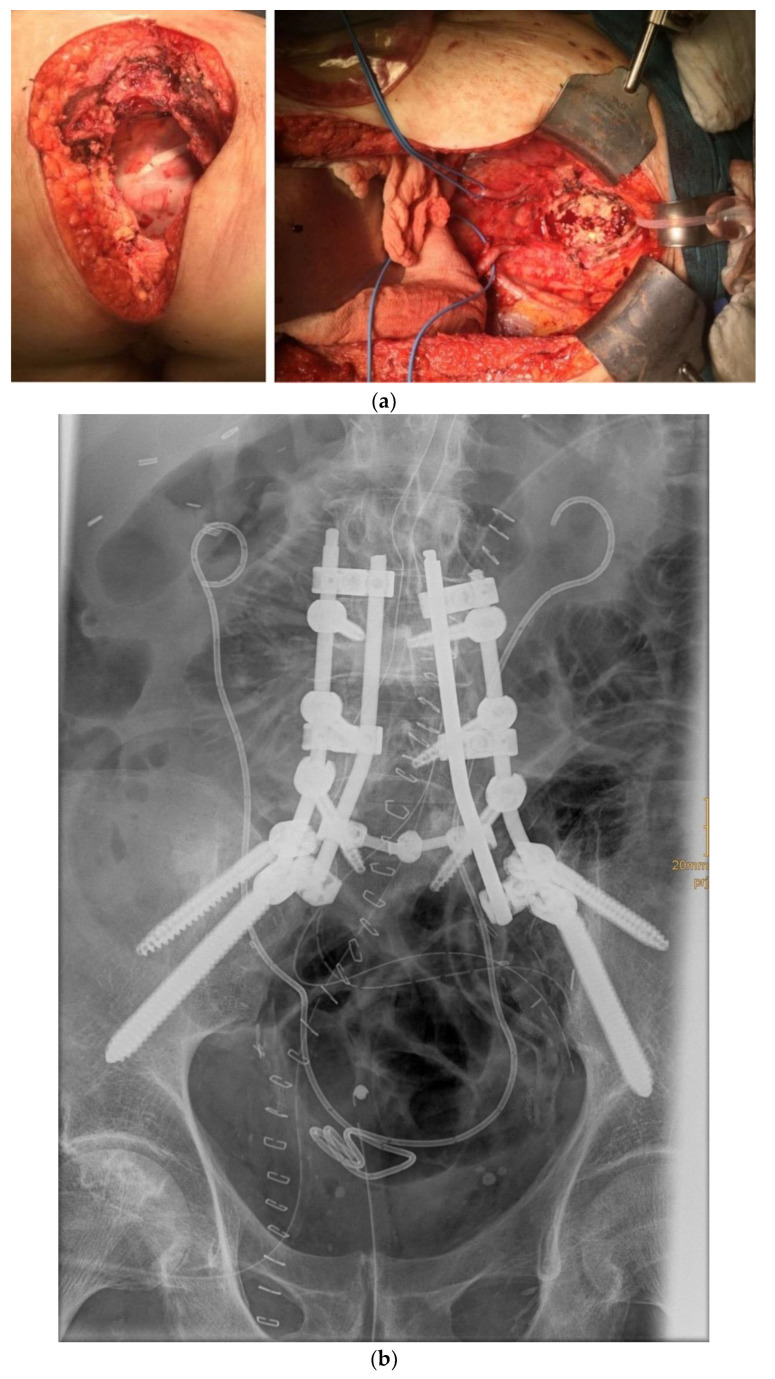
(a) Recurrent rectal carcinoma with infiltration of the os sacrum (surgeons: B. Lampe and D. Frank), (b) Recurrent rectal carcinoma with infiltration of the os sacrum; postoperative X-ray overview after stabilisation (surgeons: J. Weitz/K-D. Schaser).
